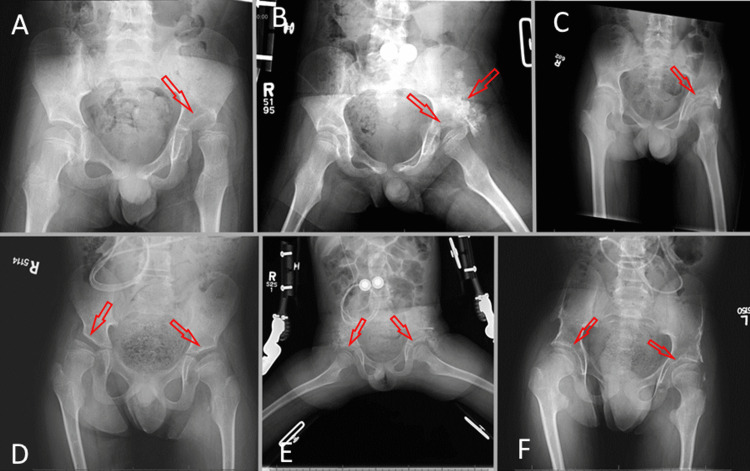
Sample radiographs. A: Patient 1, preoperative. B: Patient 1, first postoperative X-ray, left-side surgery. C: Patient 1, final postoperative X-ray. D: Patient 2, preoperative. E: Patient 2, first postoperative X-ray, bilateral surgery. F: Patient 2, final postoperative X-ray. Note: Some patients have more prominent superior lateral outcropping bone at final follow-up than others. We have not detected an association between outcomes and X-ray persistence of superior lateral bone.
